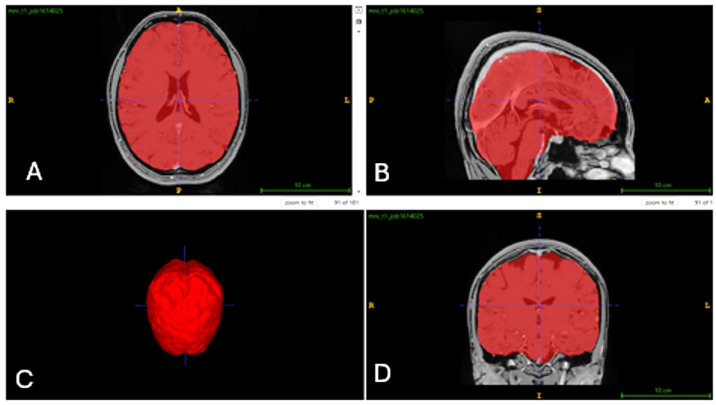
Brain volumetric analysis and 3D reconstruction on MRI scans. ((A): axial, (B): sagittal, (C): 3D image, (D): coronal).
